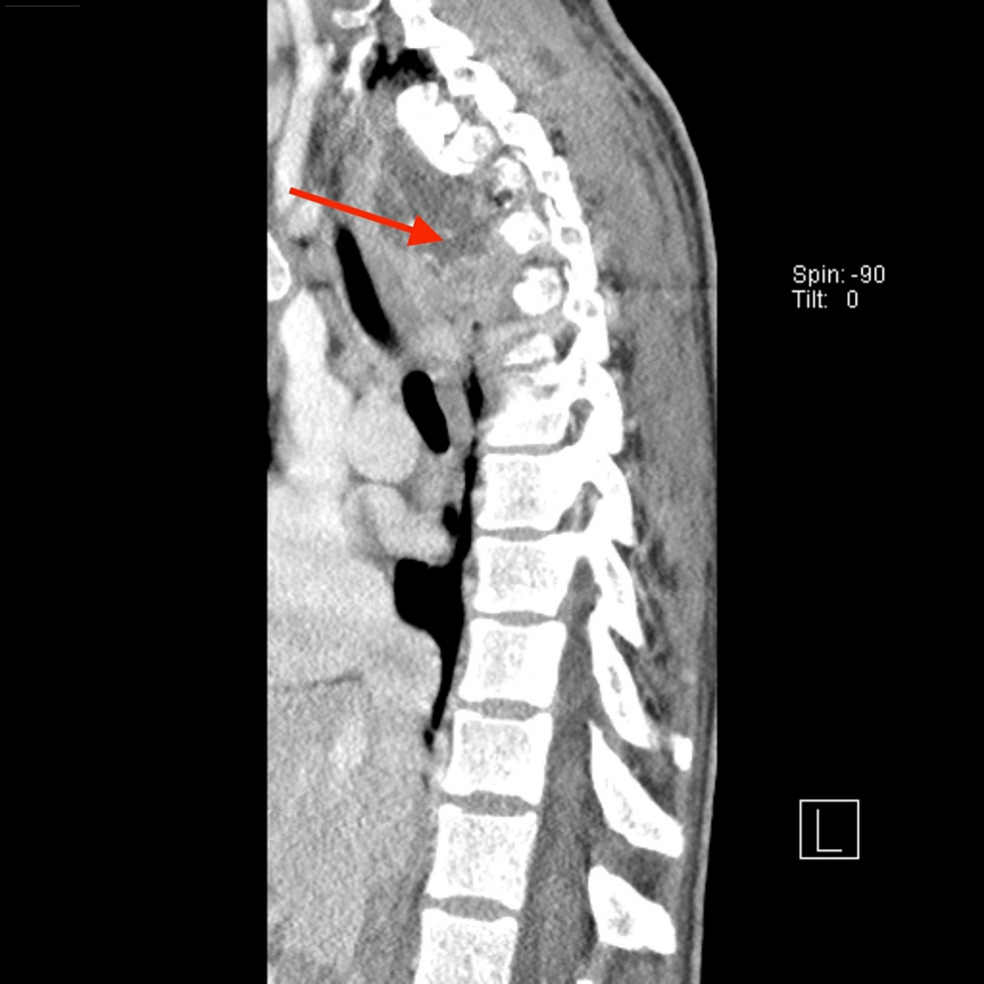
CT of the thoracic spine in a sagittal plane Sclerosis and erosive changes of T1, T2, and T4 can be noted. Associated with these bony abnormalities is a large paraspinal abscess that extends from the T1 level through the T5 level with measurements of approximately 7.5 cm in diameter with an anterior-posterior dimension of 3.3 cm, likely causing a mass effect upon the thoracic cord.
